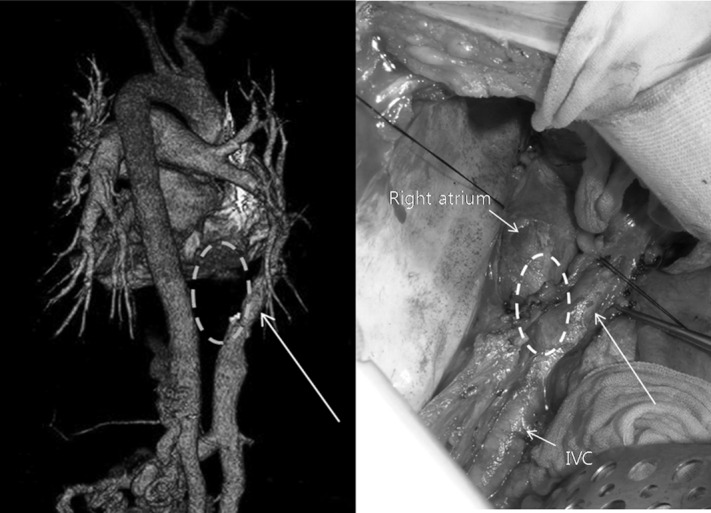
A reconstructed computed tomography (CT) angiography and intraoperative findings showed interruption of IVC (white circle), and anomalous right pulmonary vein (white arrow) drained into the IVC.
